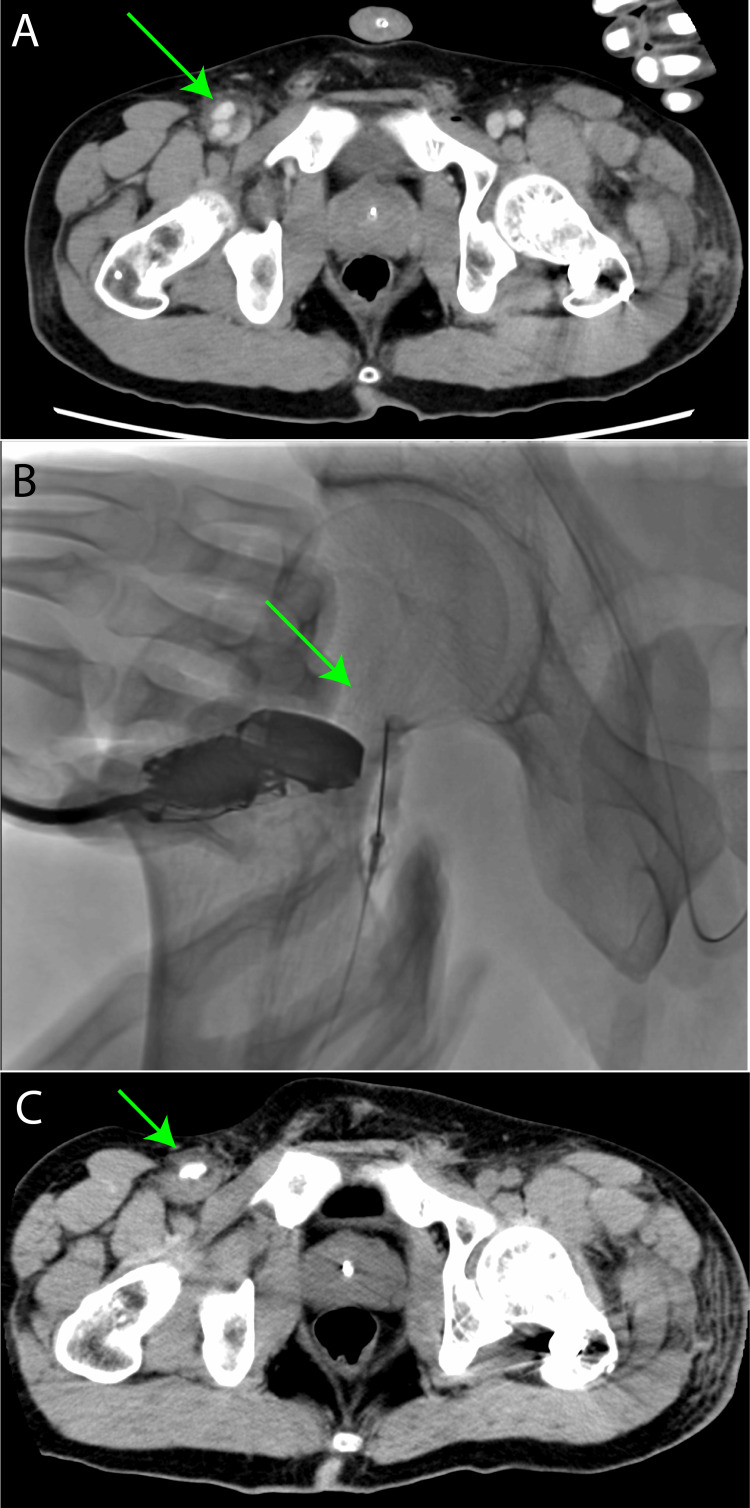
Imaging on postinjury day 3. (A) Contrast-enhanced CT showed the presence of a right femoral artery pseudoaneurysm (green arrow). (B) Thrombin was injected into the pseudoaneurysm in the angiography suite under ultrasound guidance (green arrow). (C) The contrast agent used during the procedure remained within the pseudoaneurysm (green arrow). CT: computed tomography
